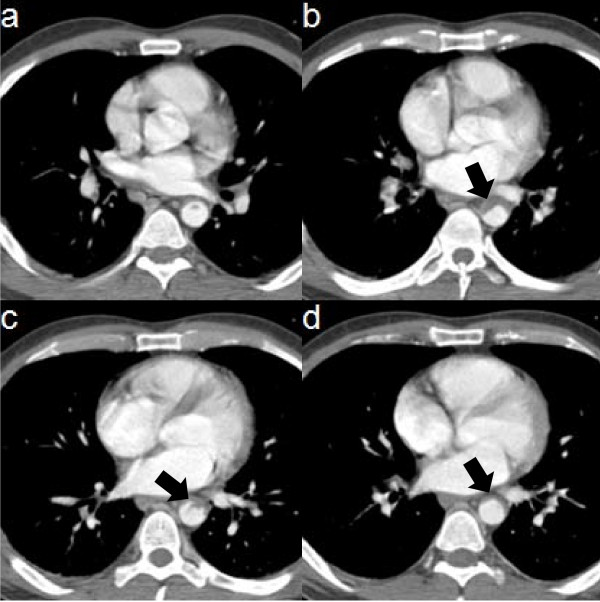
CT images demonstrating the occlusive thrombus in the descending thoracic aorta (black arrows).
